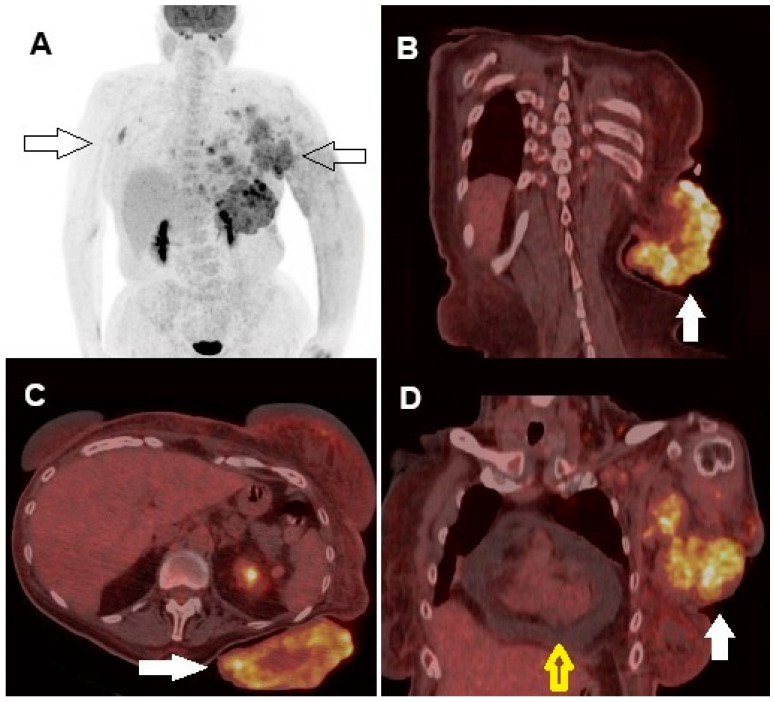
Whole-body [^18^F]FDG PET/CT showed increased glucose metabolism in the cutaneous and subcutaneous tissue surrounding the tumors, as well as in several lymph node stations (right axillary lymph nodes and, on the left side, axillary, supraclavicular, and along the internal mammary chain) ((A), arrows). High tracer uptake in the cutaneous lesion of the left scapula was clearly visible in the fused coronal ((B), arrow) and axial ((C), arrow) views, as well as in the fused coronal slice of the left breast region ((D), arrow). Furthermore, coronal images showed pericardial effusion ((D), yellow bordered arrow). Quantitative PET-derived parameters for the largest scapular region were as follows: maximum standardized uptake value (SUVmax), 10.4; metabolic tumor volume (MTV), 52 cc; and total lesion glycolysis (TLG), 267 g/mL. No visceral or skeletal metastases were detected. Subsequent cerebral magnetic resonance imaging (MRI) did not identify any brain metastases. According to the 8th edition of the AJCC TNM Classification, the disease stage was determined to be T4bN3cM1a0, corresponding to stage IV [7]. Given the locally advanced and large primary tumor along with the presence of multiple metastatic lymphadenopathies, we opted for neoadjuvant systemic treatment. Our aim was to reduce the tumor size to make resection feasible, thereby potentially improving the patient’s quality of life. The patient underwent combined targeted therapy with BRAF and MEK inhibitors: Dabrafenib 150 mg b.i.d. plus Trametinib 2 mg q.d. After only one week, the outcome was remarkable, with partial regression of both primary tumor and metastases, presence of necrotic areas, and size reduction, confirmed by further regression of the lesions upon objective evaluation at 1 month.
